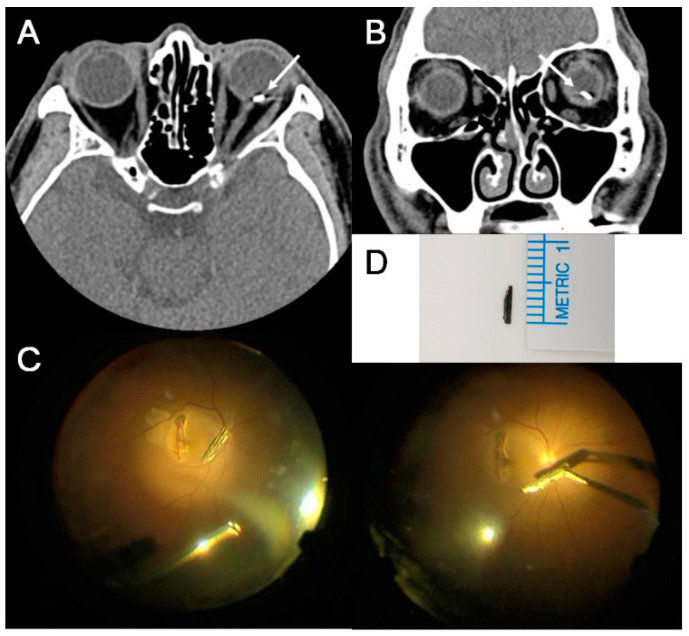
CT scan and intraoperative view of a 59-year-old male patient struck by a metallic foreign body in his left eye during work. (A,B) Axial and coronal CT images of the patient show a hyperdense foreign body (*long white arrows*) embedded in the retina. (C) Intraoperative view during vitrectomy shows a macular tear and an intraocular foreign body (IOFB). The IOFB was retrieved with vitreous forceps. (D) The length of the removed metallic foreign body is 5 mm.
